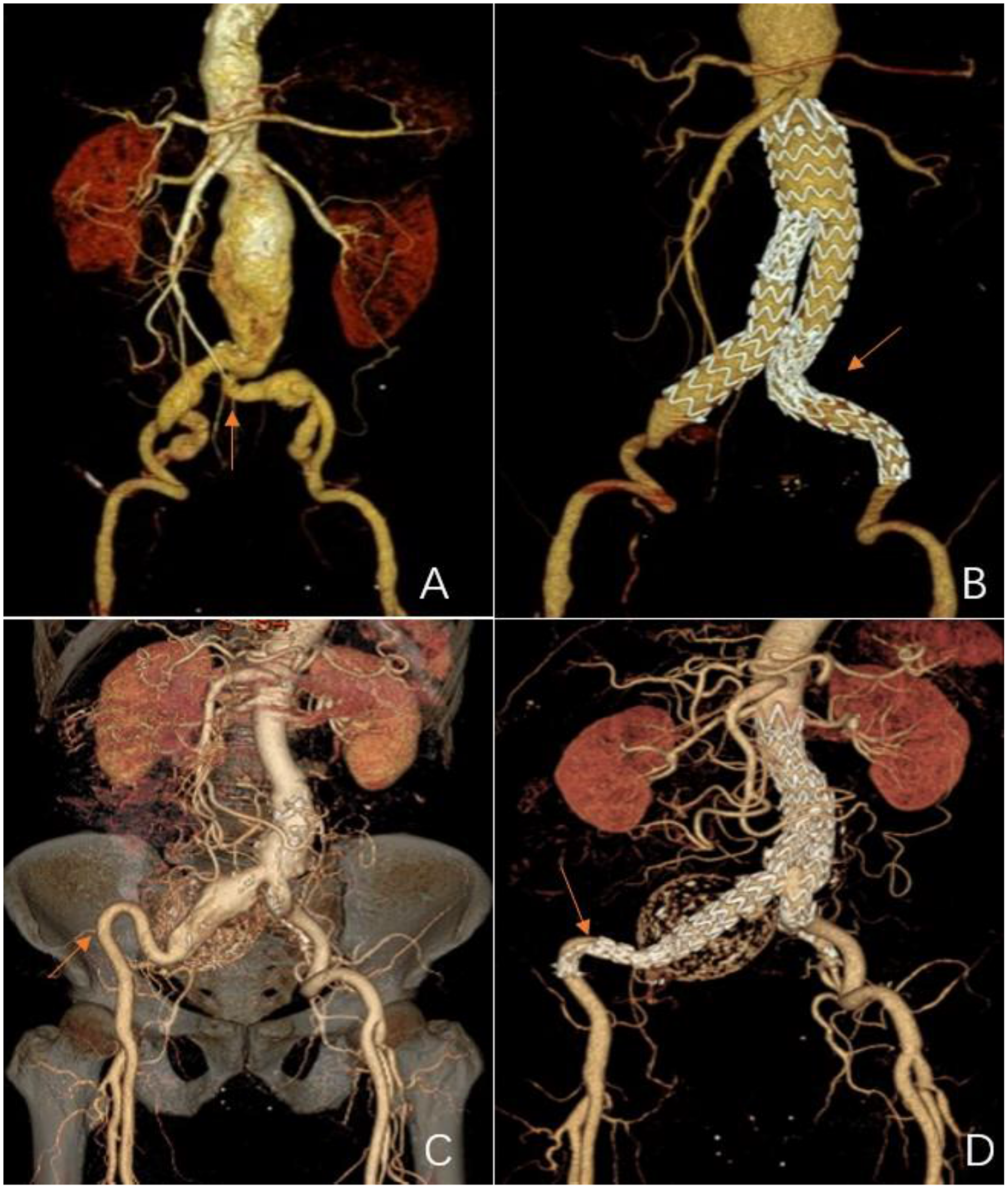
Representations of metal bare stents placed in the common or external iliac arteries. (A) and (C) Pre-operative 3D CT scans showing severe tortuosity of the left CIA (red arrow), and the double iliac sign of the right EIA (red arrow). (B) A post-operative 3D CT scan 18 months after EVAR of a bare metal stent that was placed in the left CIA (red arrow,) showing stent patency. (D) A post-operative 3D CT scan 124 months after an EVAR of the other bare metal stent that was placed in the right EIA (red arrow) showing stent patency.
